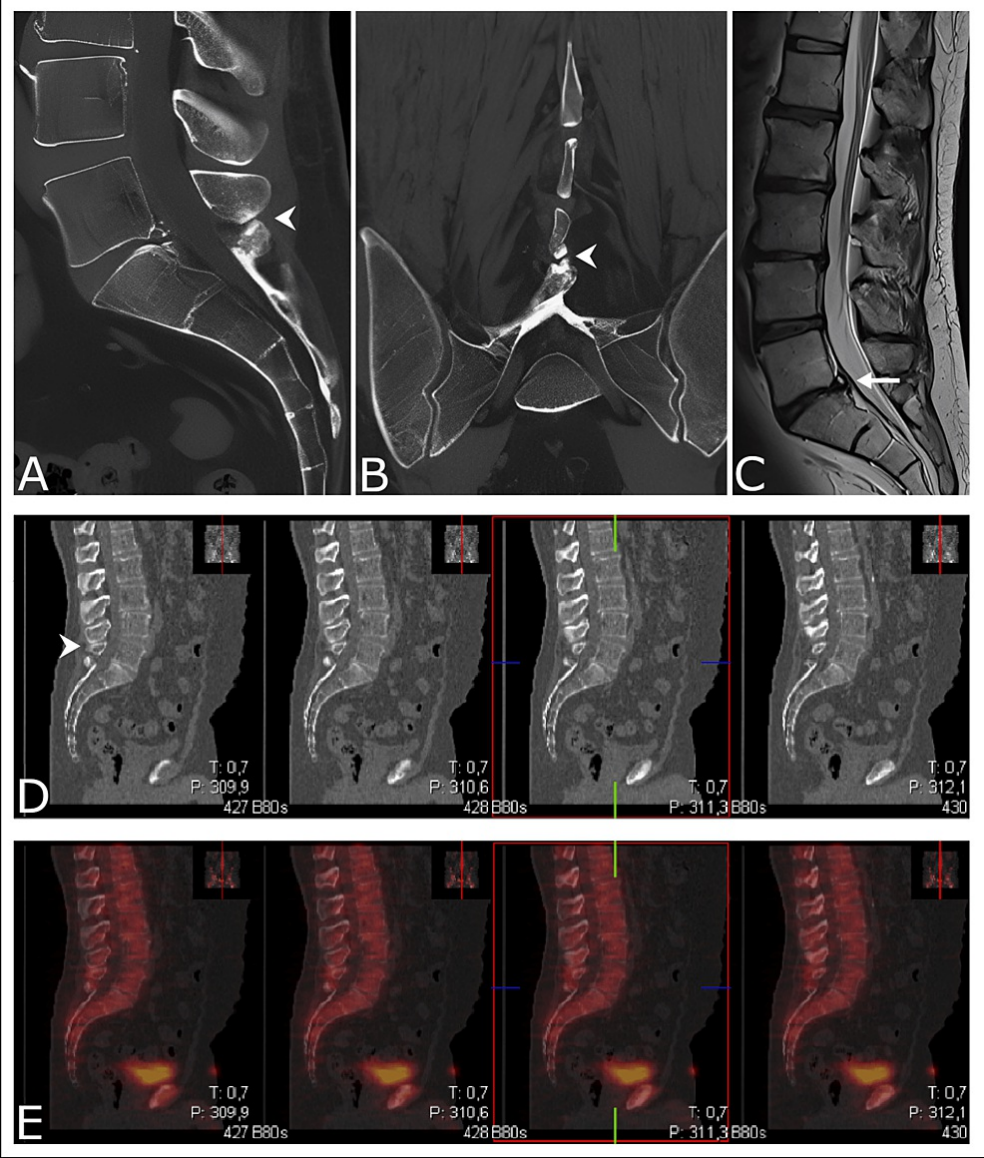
Imaging modalities for diagnostic workup in Baastrup’s disease. (A) Sagittal CT of the lumbosacral junction. The arrow indicates the close relationship of the spinous process L5 and S1 with reactive sclerosis of the opposing interspinous surfaces. A reduced interspinous space of spinous processes L4-L5 is observed. (B) Corresponding coronal CT. The arrow indicates the approximation and contact of the adjacent spinous processes of L5 and S1. (C) T2-weighted sagittal MRI of the lumbosacral junction. Minor degenerative changes and postoperative changes at the L5-S1 segment (long arrow) are evident. (D) The contact of the L4 and L5 spinous processes is visible (arrow). (E) SPECT data do not demonstrate any relevant tracer uptake. CT = computed tomography; MRI = magnetic resonance imaging; SPECT = single-photon emission computed tomography
